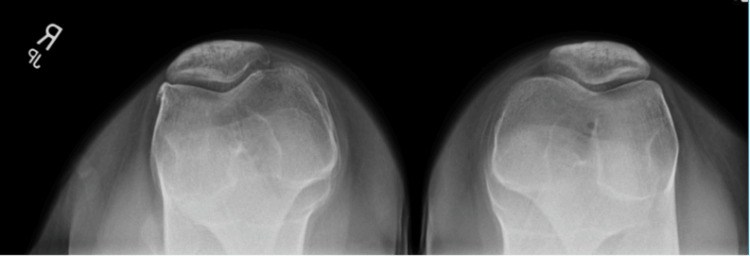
Bilateral axial (sunrise) radiographs of the patellofemoral joints showing no acute fractures
